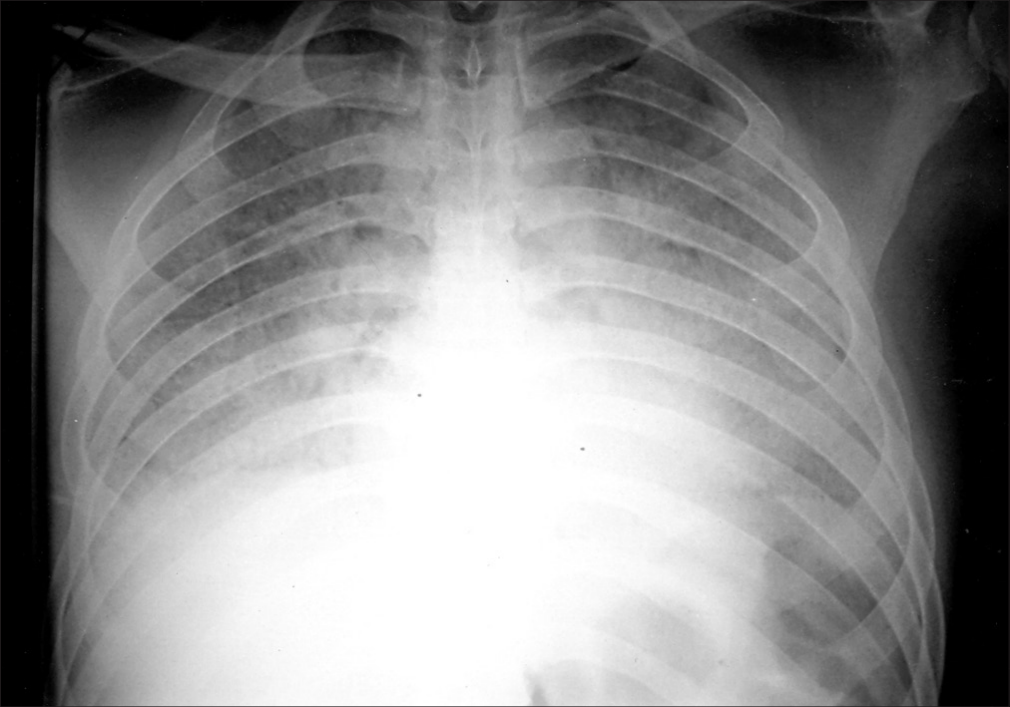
Chest radiograph showing bilateral diffuse reticulonodular
